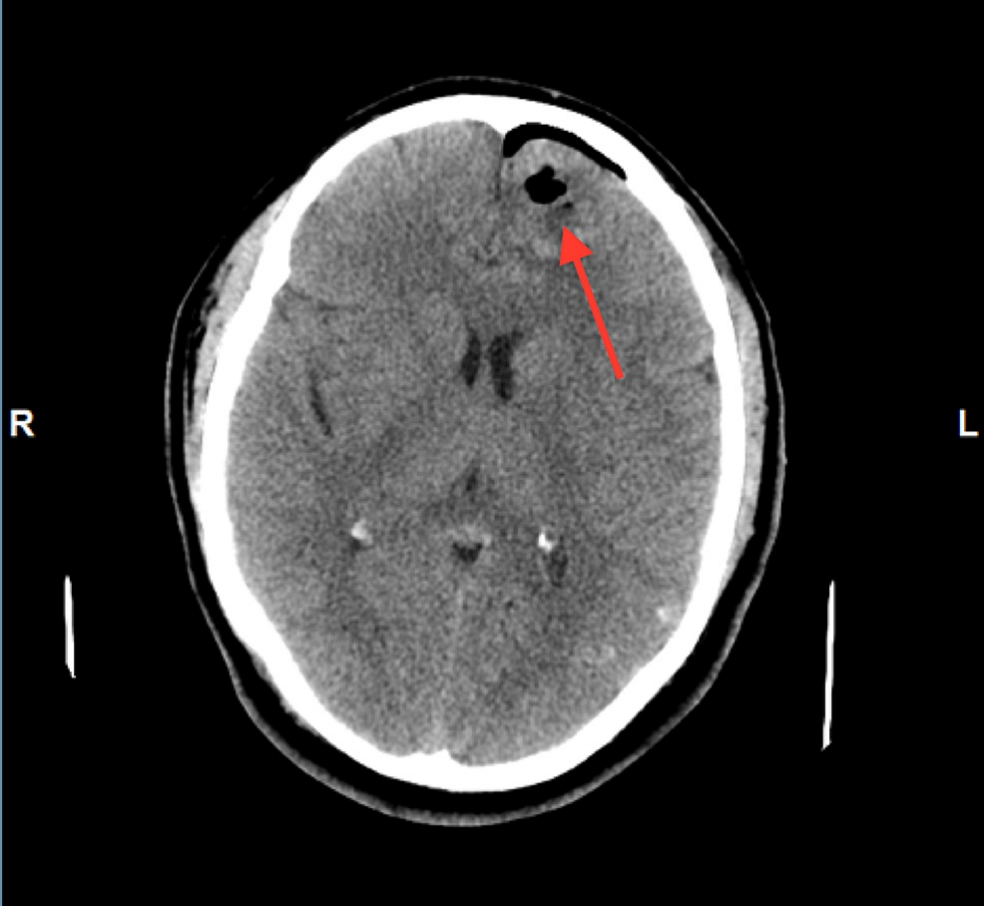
CT head after excisional biopsy CT: computed tomography
